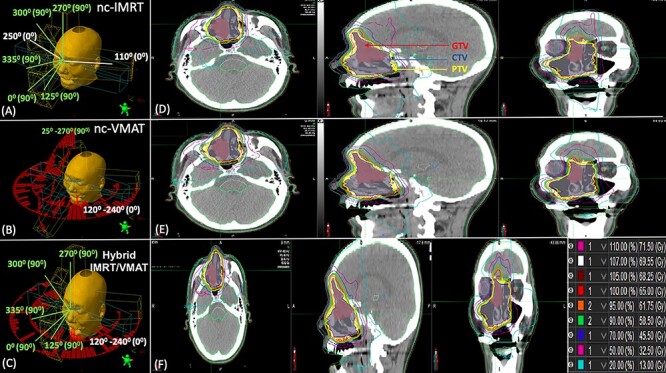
Overview of the beam configuration and dose distribution on axial, sagittal and coronal CT slices of the non-coplanar IMRT (A and D), non-coplanar VMAT (B and E) and Hybrid IMRT/VMAT (C and F) treatment plan. The beam direction range and couch angle in all patients is given per beam direction. The coplanar beam is marked in white, and the non-coplanar beam direction is marked in green.
